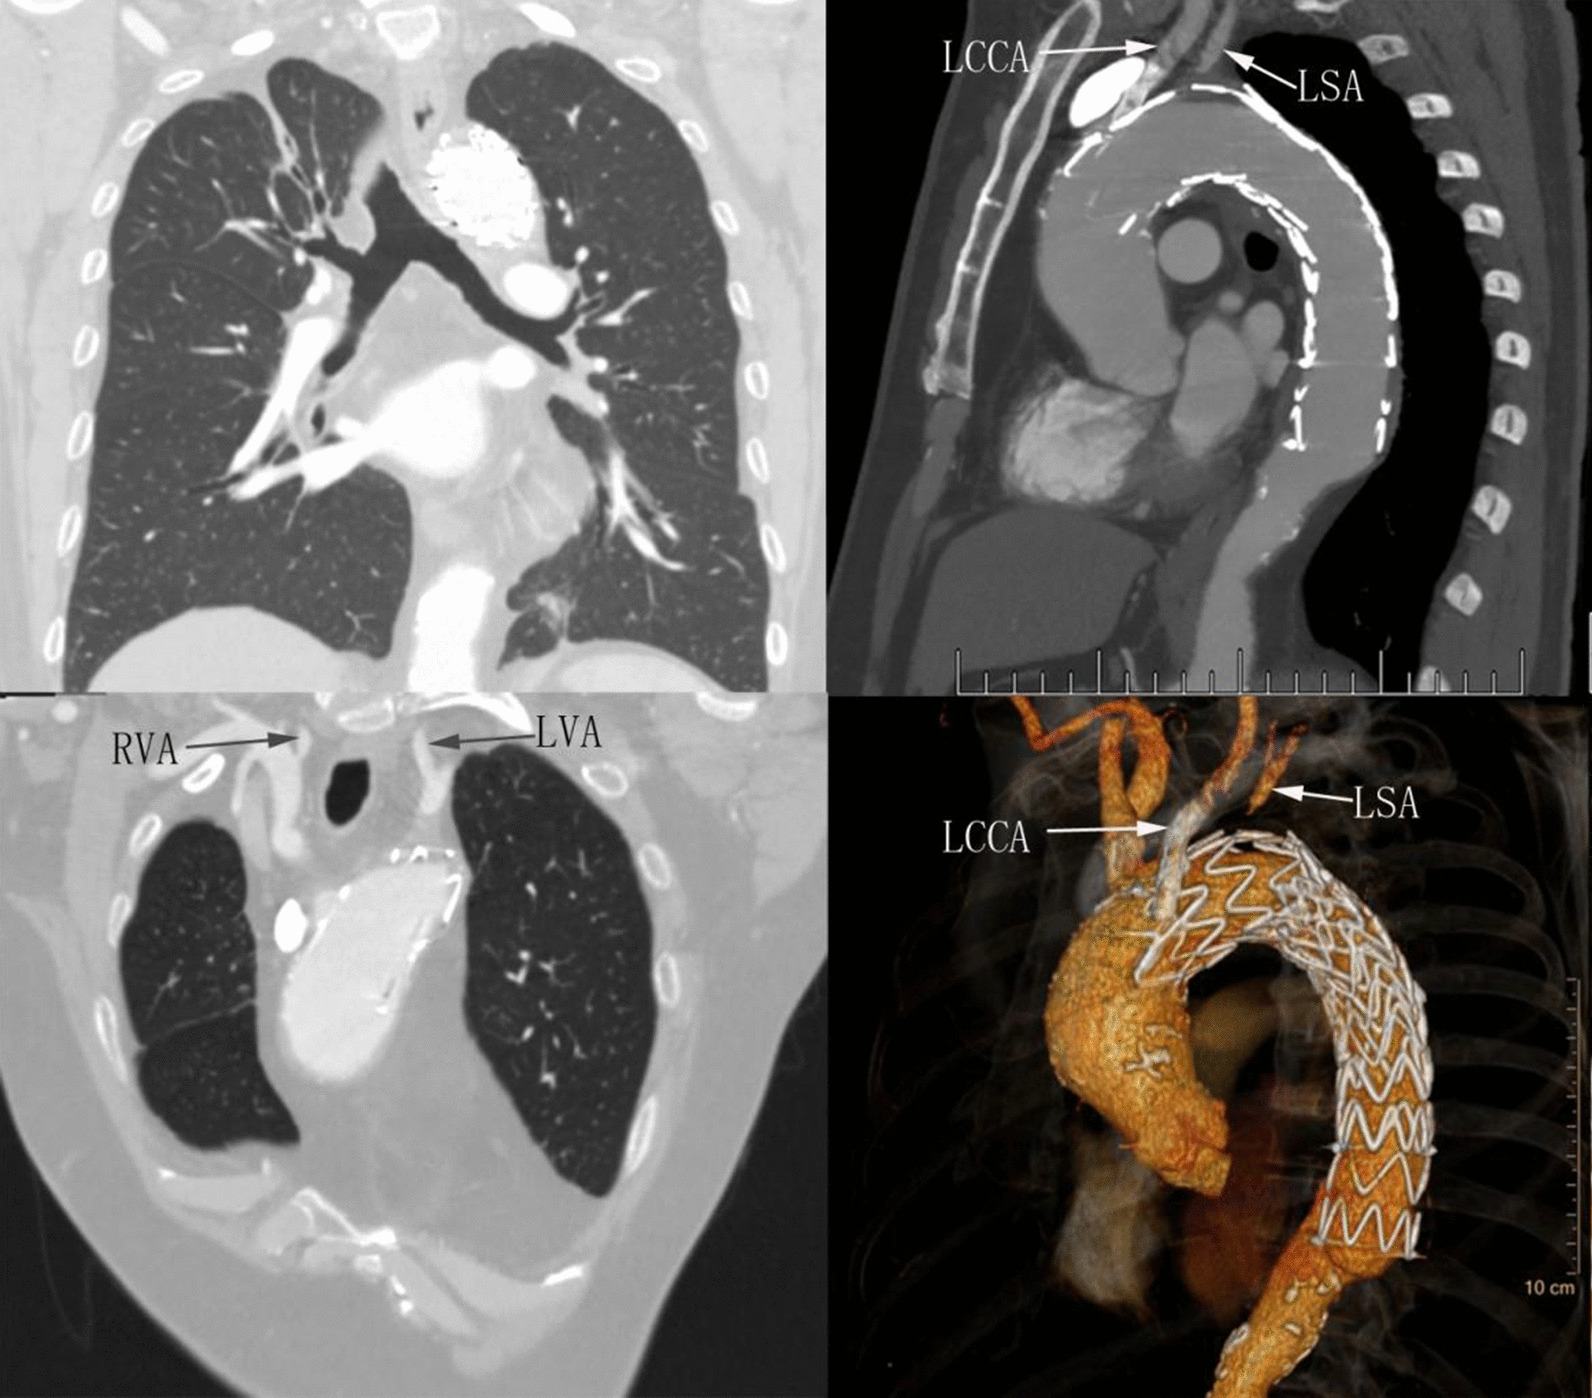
Postoperative CTA confirmed complete regression of the aortic-arch pseudoaneurysm, no bronchial stenosis, the patency of chimney stent and left subclavian artery. RVA: right vertebral artery, LVA: left vertebral artery, LSA: left subclavian artery, LCCA: left common carotid artery
